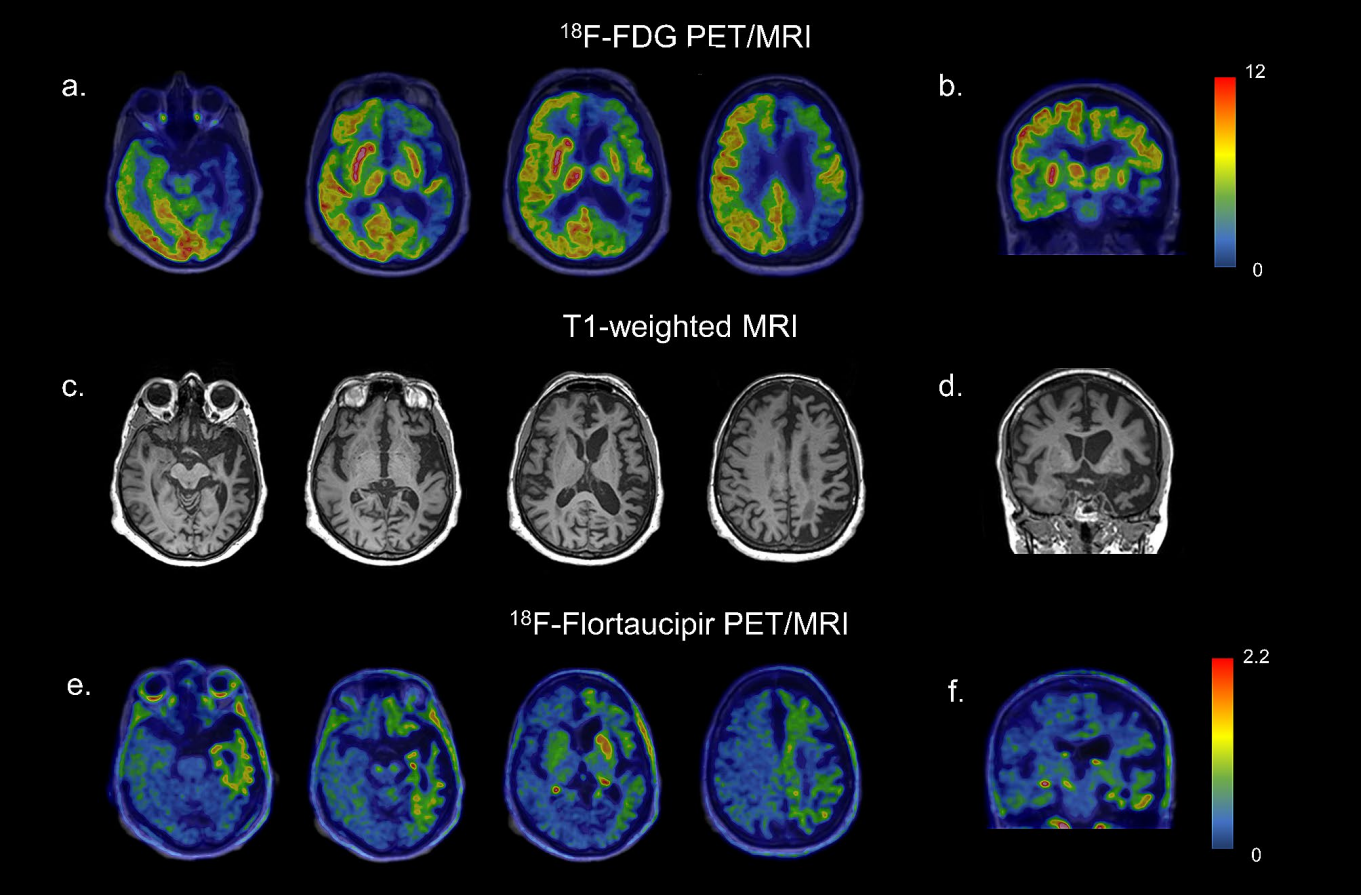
Axial (a) and coronal (b) slices of [^18^F]FDG PET showing severe asymmetric hypometabolism in the left frontotemporoparietal cortex and in the left caudate nucleus and in the left putamen. Axial (c) and coronal (d) T1-weighted MRI images show supra-tentorial asymmetric brain atrophy, predominating on the left side. Axial (e) and coronal (f) [^18^F]flortaucipir PET images show a mildly increased tracer uptake in areas characterized by hypometabolism.Images are in radiological orientation
